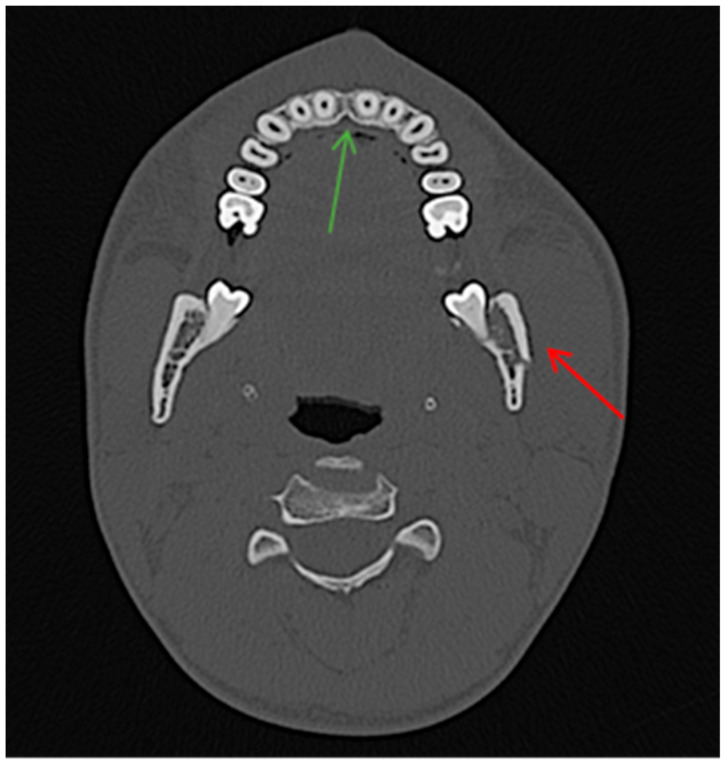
A 19-year-old male patient diagnosed with a displaced fracture of the left angle of the mandible with an impacted tooth in the fracture gap (red arrow). CT was performed 2 days after the injury. No air was observed in the region of the fracture. In the region of the anterior teeth, from the lingual side, the presence of air bubbles associated with saliva is observed (green arrow).
